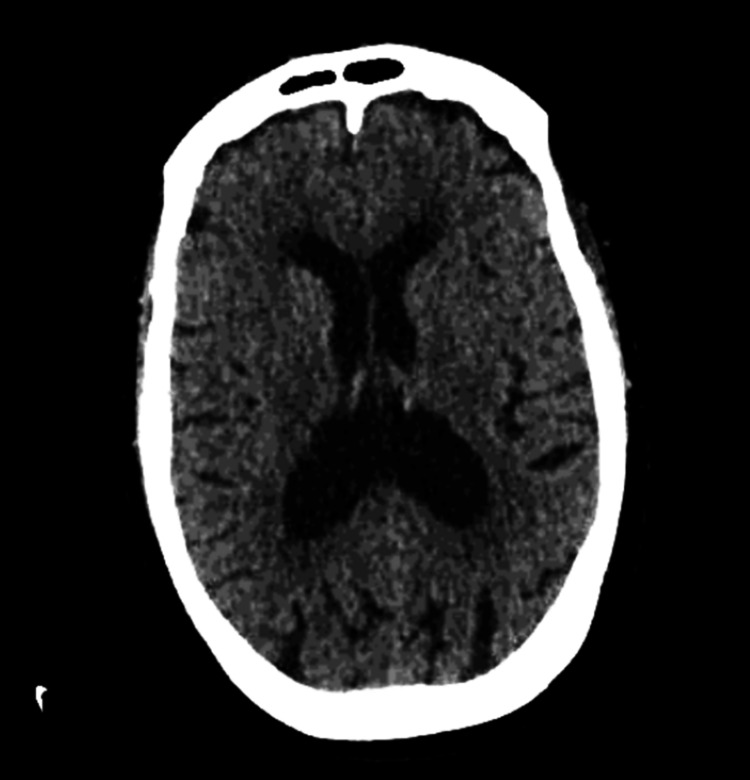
CT head showing no acute intracranial abnormalities
